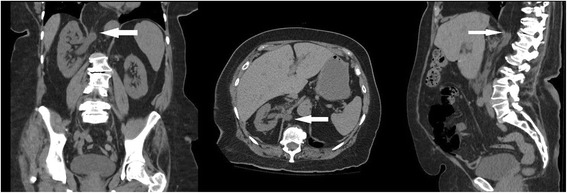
Patient’s initial computed tomography (CT) at presentation demonstrating small loop of ureter within right-sided diaphragmatic hernia. Coronal, Axial, Sagittal views with white arrow showing segment of herniated ureter
